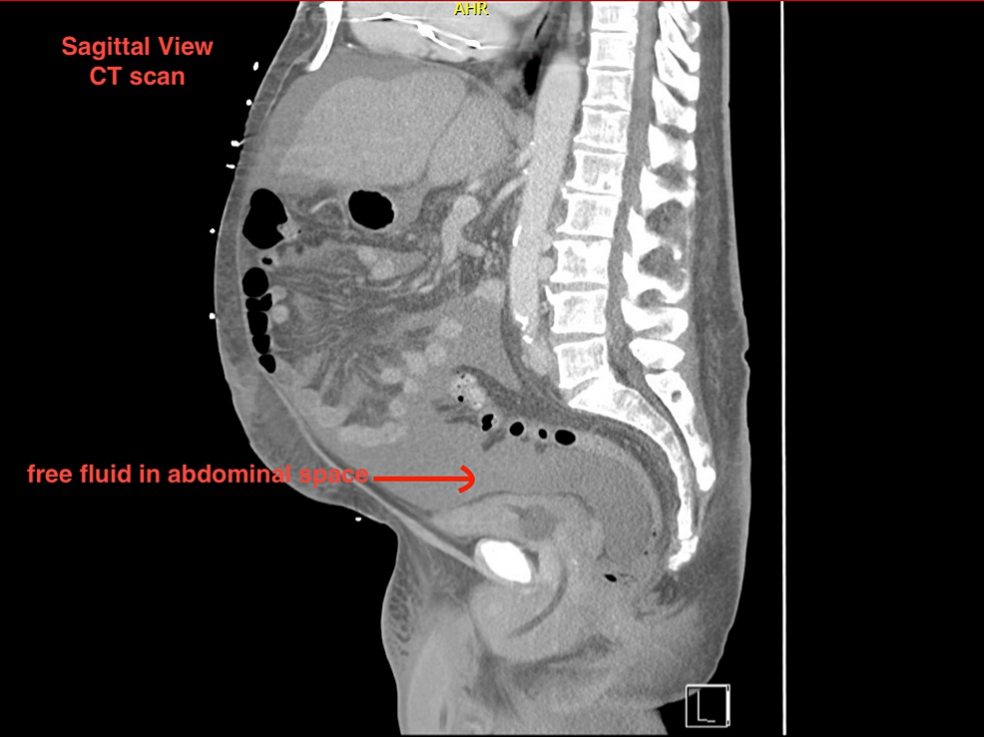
Sagittal view of abdominal CT scan The red arrow indicates free fluid in abdominal fluid. IVC thrombus is not visualized in this view, refer to Figure 2 for an additional view. IVC: Inferior vena cava
